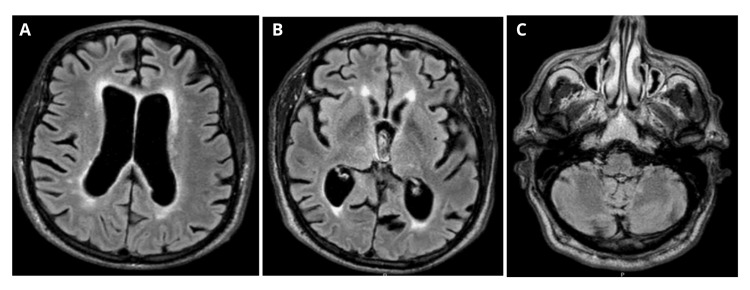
Brain MRI showing subcortical atrophy and white matter lesions due to small-vessel disease in T2/FLAIR (A-C) FLAIR: Fluid-attenuated inversion recovery
